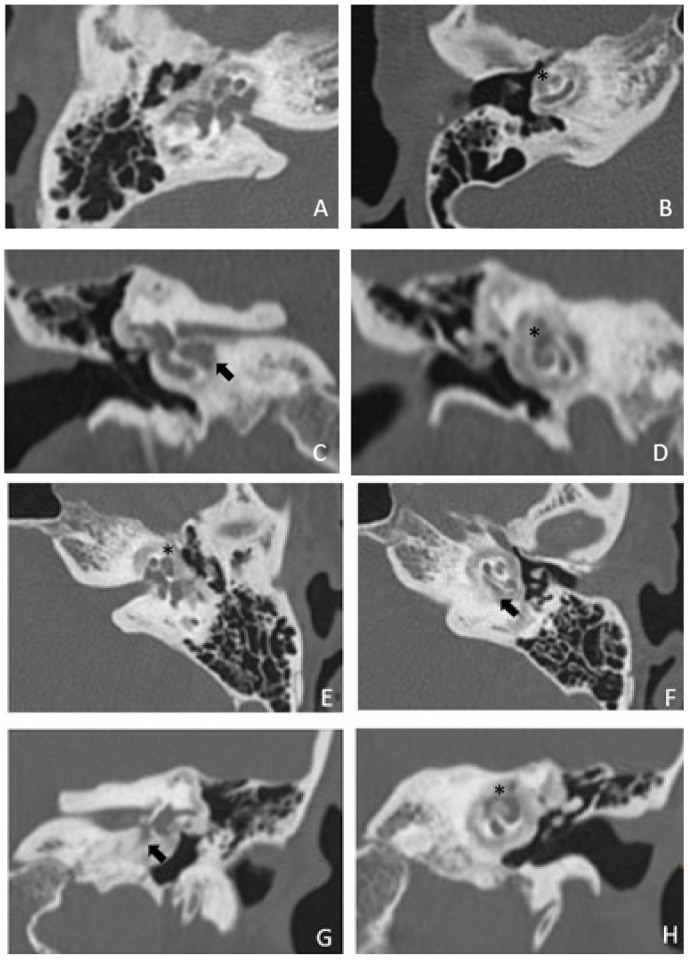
CT images of patient #1 with osteogenesis Imperfecta. (A,B), right side in axial plane. (C,D), right side in coronal plane. (E,F), left side in axial plane. (G,H), left side in coronal plane. A pericochlear cavity is visible in all figures; arrows indicate its origin from the IAC. Asterisks indicate extension of osteorarefation into the cochlea.
